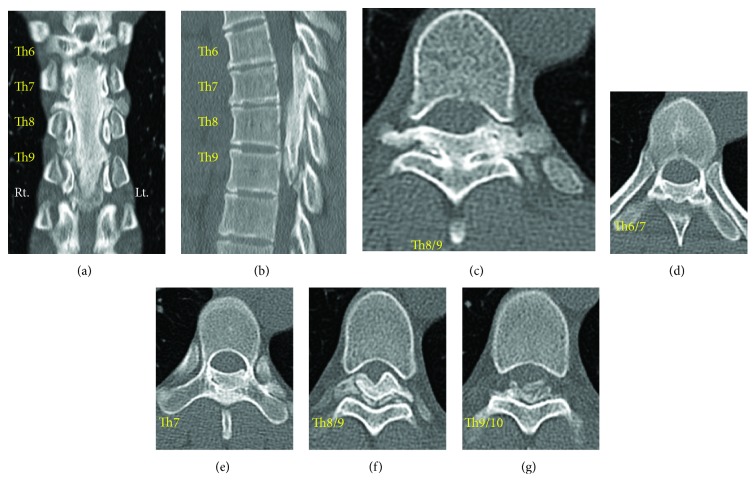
Preoperative thoracic CT. Ossified epidural posterior mass compressing the thoracic cord between Th6 and Th9 was revealed in coronal view (a) and sagittal view (b) of thoracic CT. Ossified lesion reached to extraforamen at Th7/8 intervertebral levels (c). Axial view of epidural ossified mass in Th6/7 (d), Th7 (e), Th8/9 (f), and Th9/10 (g) levels, which occupied about 50-80% of the spinal canal.
